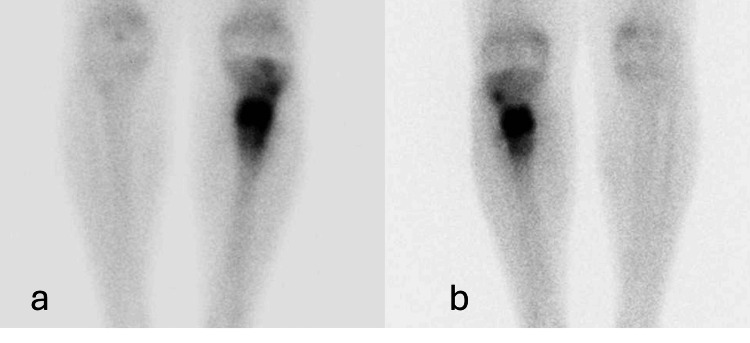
Bone scintigraphy with 99mTc-MDP, static bone tracing phase (a) Anterior view of static bone tracing phase with 99mTc-MDP in the lower limbs. (b) Posterior view of static bone tracing phase with 99mTc-MDP in the lower limbs.
